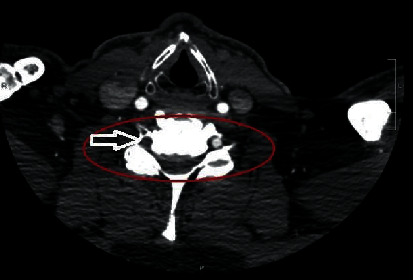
The computed tomography angiography (CTA) of the the head and neck showed right vertebral artery occlusion with moderate diffuse intracranial atherosclerosis.
